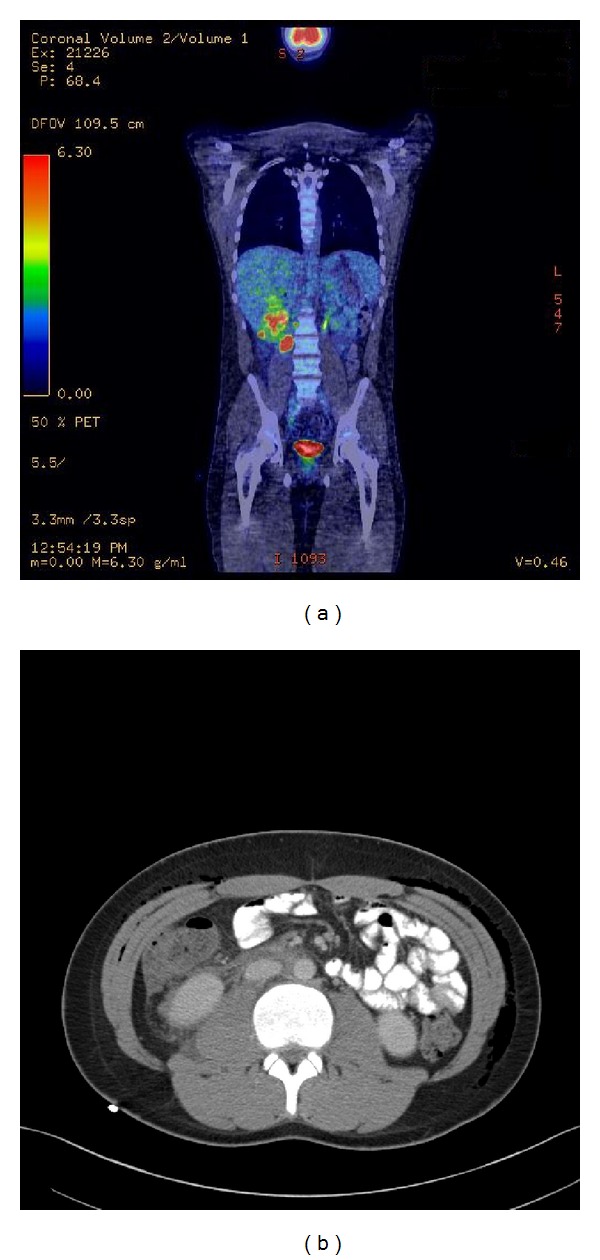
Coronal PET-CT and contrast CT images demonstrating PET-avid areas suspicious for lymphoma and vague infiltrative soft tissue process in the retroperitoneum.
